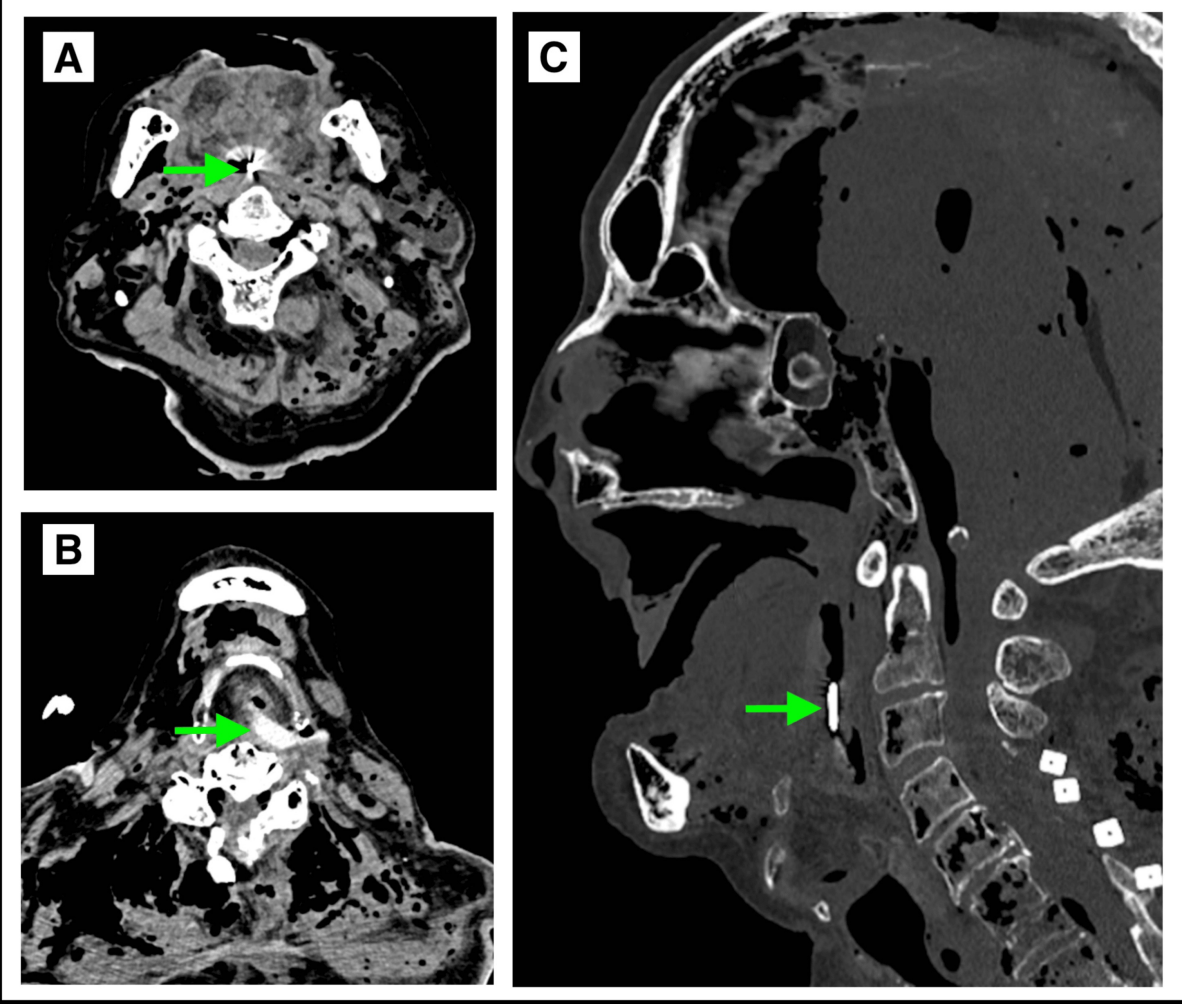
Postmortem CT findings (A) Axial image at the level of the second cervical vertebra (C2) showing a punctate hyperdense area with imaging artifact (green arrow).
